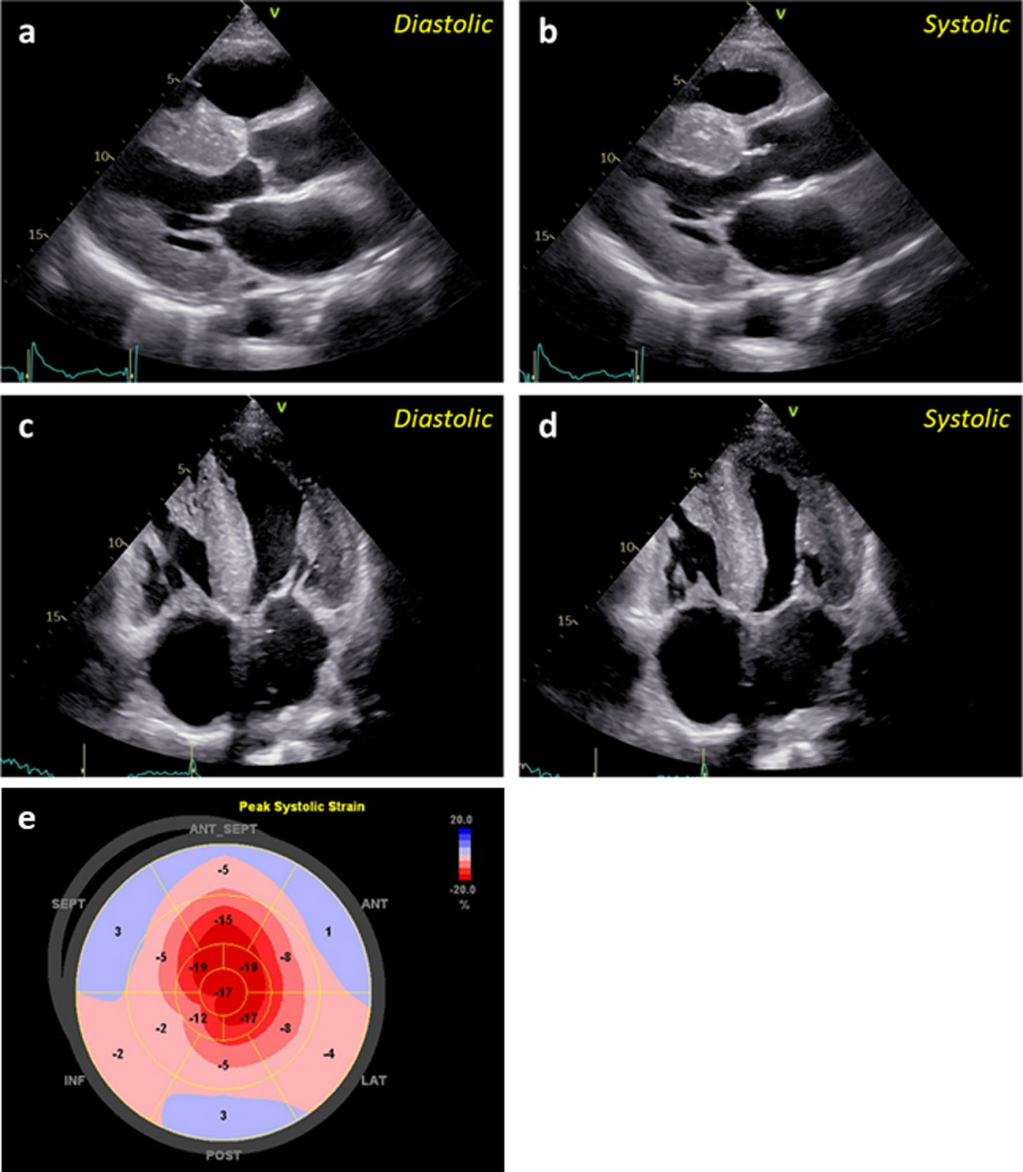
Diffuse cardiac hypertrophy and apical sparing pattern on transthoracic echocardiography. Symmetric left ventricular hypertrophy and mild systolic dysfunction are observed in the parasternal long axis view (a, b) and four-chamber view (c, d). Two-dimensional speckle-tracking echocardiography shows a reduced longitudinal strain in the basal segments and preserved longitudinal strain in the apical segments of the left ventricular myocardium (apical sparing) (e)
